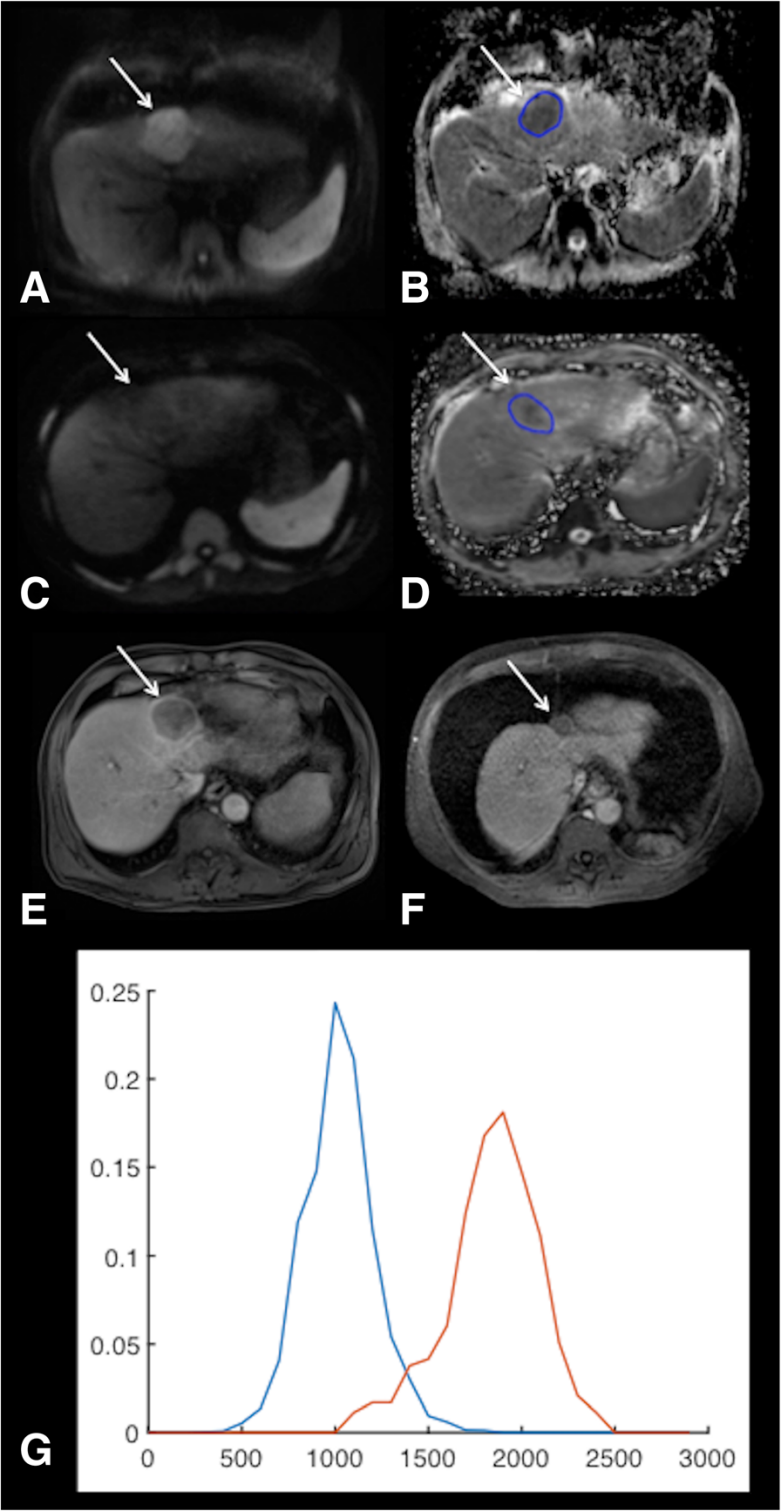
63-year-old male patient with HCV cirrhosis and HCC treated with radioembolization (RE). Pre-treatment MRI: HCC (arrows) demonstrates restricted diffusion with high signal on high b value DWI (b 800) (a) and low ADC on ADC map (b). Post-treatment MRI at 6w post RE shows resolution of diffusion restriction on DWI (b 800) (c) with residual low ADC areas in tumor (d). Contrast-enhanced-T1weighted images obtained during the portal-venous phase at baseline (e) and 6 months (f) show complete tumor response at 6 months. Corresponding histogram distribution of vADC at baseline (blue, vADC median 1.08 × 10^− 3^ mm^2^/s, vADC mean 1.07 × 10^− 3^ mm^2^/s) and 6w after RE (red, vADC median 1.91 × 10^− 3^ mm^2^/s, vADC mean 1.89 × 10^− 3^ mm^2^/s, ΔvADC median 78%, ΔvADC mean 76%) shows a shift of the distribution to the right after RE (g)
